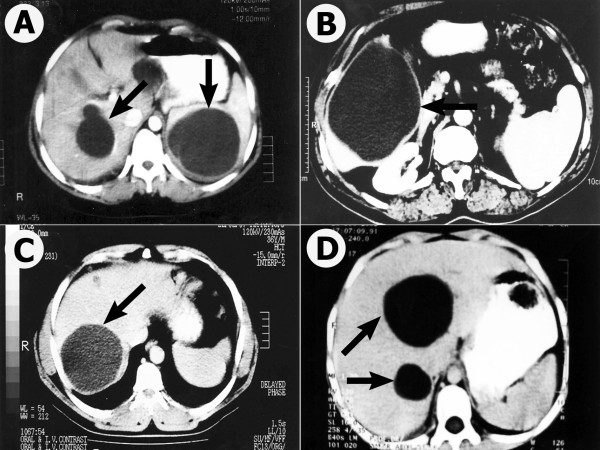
Photographs of transverse computed tomography scan through the liver showing cystic hydatid lesions. Figs. A, B, C, and D refer to case one to four, respectively. Arrows indicate the hydatid cyst.
